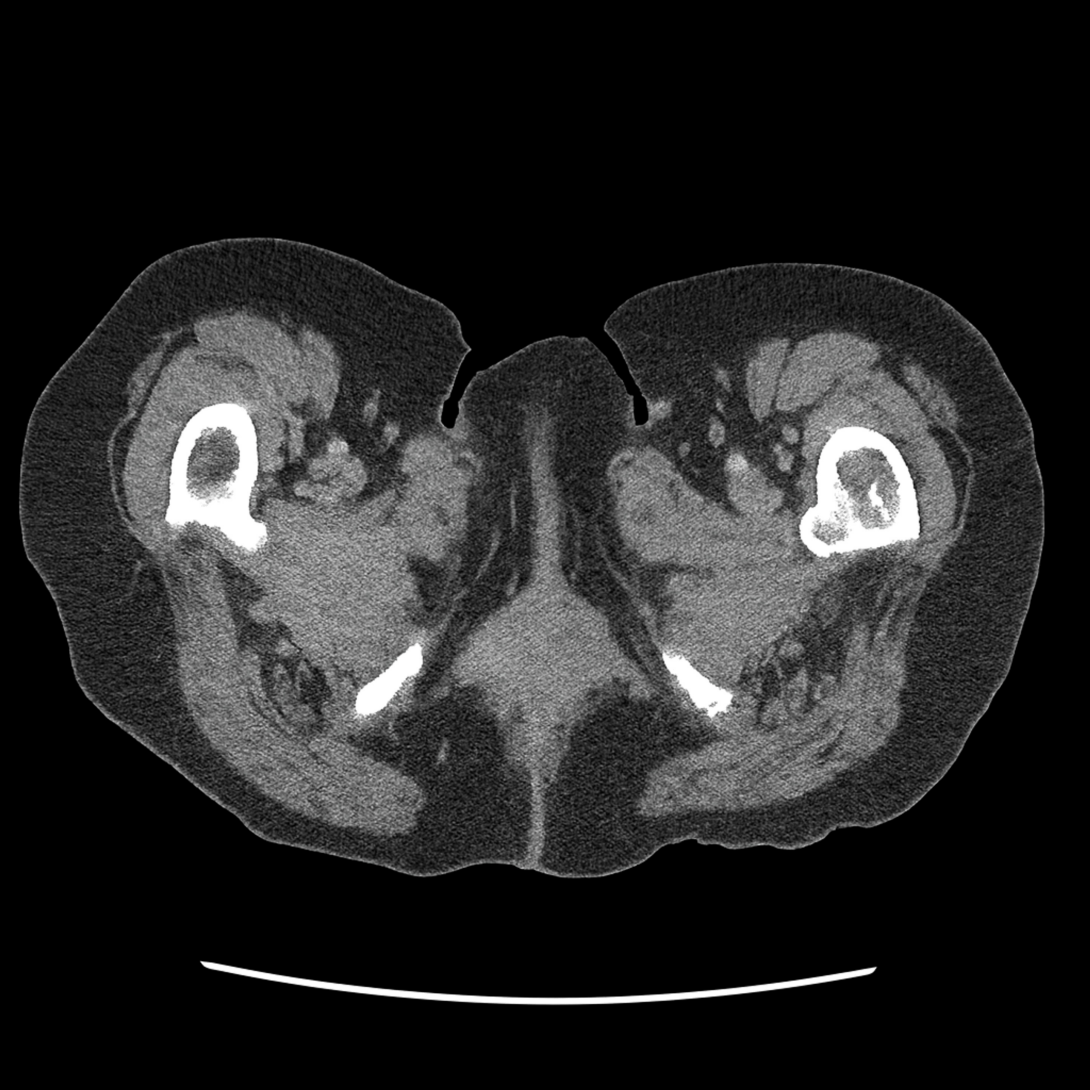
Contrast-enhanced CT abdomen and pelvis demonstrating a hyperdense mass arising from the right lateral vaginal wall.
